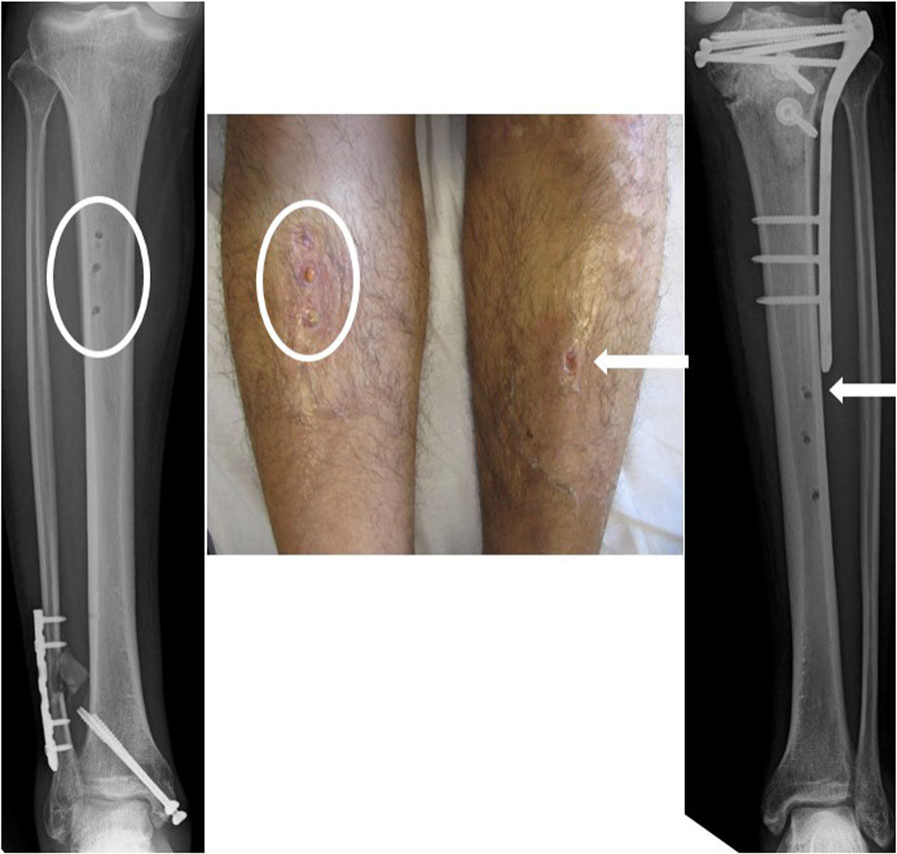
Anteroposterior radiography and physical appearance of both *lower legs*. There was discharge of pus from the bilateral tibial pin tracts (*encircled in white* on the *right tibia* and shown by *white arrows* on the *left tibia*)
